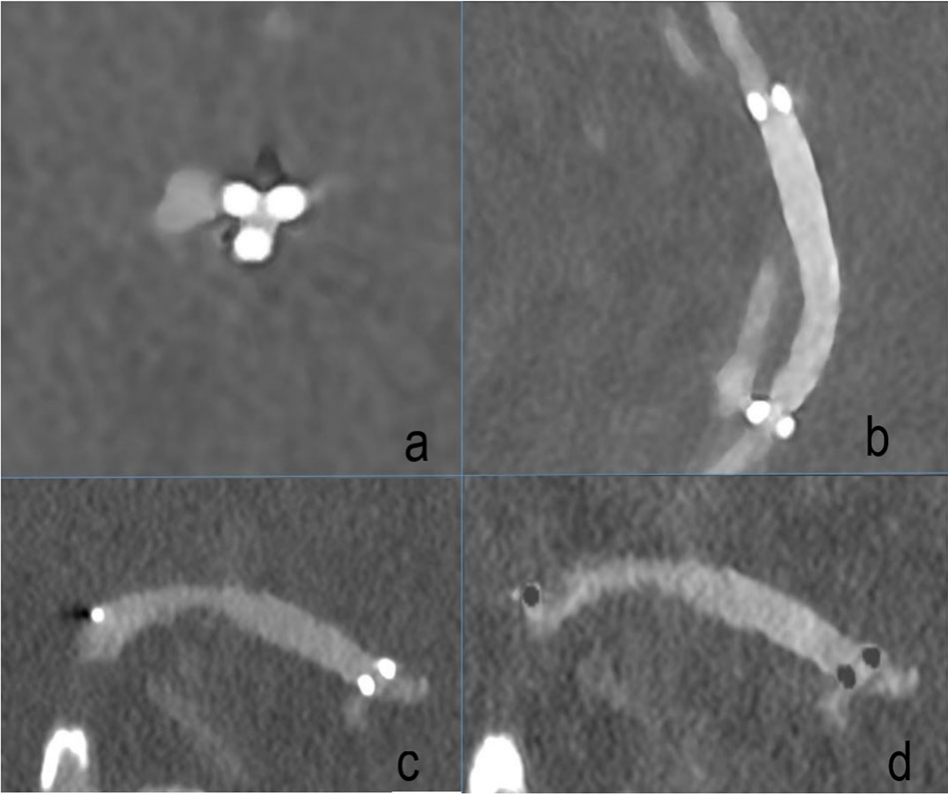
Limitations of photon-counting computed tomography angiography to overcome device-related artifacts. a Orthograde reconstruction of a stent at the level of the distal stent marking in the A3 segment of the left anterior cerebral artery. b Longitudinal section through the stent in the said vessel segment. c Virtual monoenergetic reconstruction (Bv72, 40 keV) and (d) Iodine reconstruction (Bv 72) in coronal orientation of a stent in the left middle cerebral artery. The distal stent markers at the left edge of the image are located at the transition to the proximal M2 segment. Bv, Body vascular kernel
